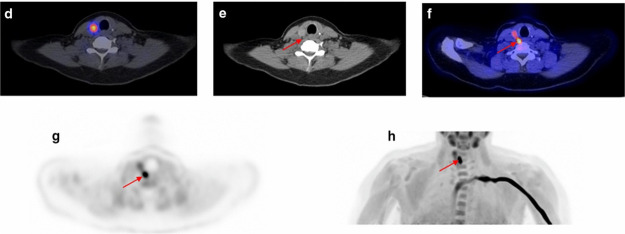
[^11^C]Choline axial PET (d), CT (e), PET/CT
